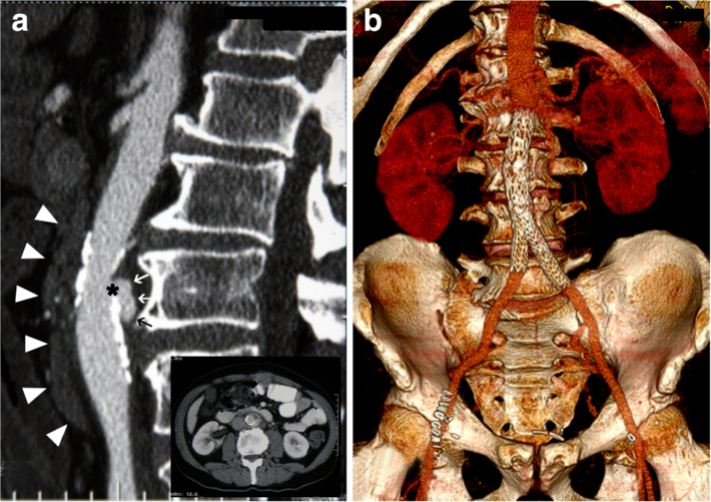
(a) Sagittal reconstruction of the contrast-enhanced initial computed tomography (CT) scan showing typical appearance of a mycotic aneurysm: eccentric saccular pseudo-aneurysm (arrows) of the abdominal aorta (arising from a ‘breach’(*) in the aortic wall) and periaortic soft tissue stranding (arrowheads). (b) 3D-reconstruction of the postinterventional computed tomography (CT) scan showing the anatomic location of the aortic stent graft.
